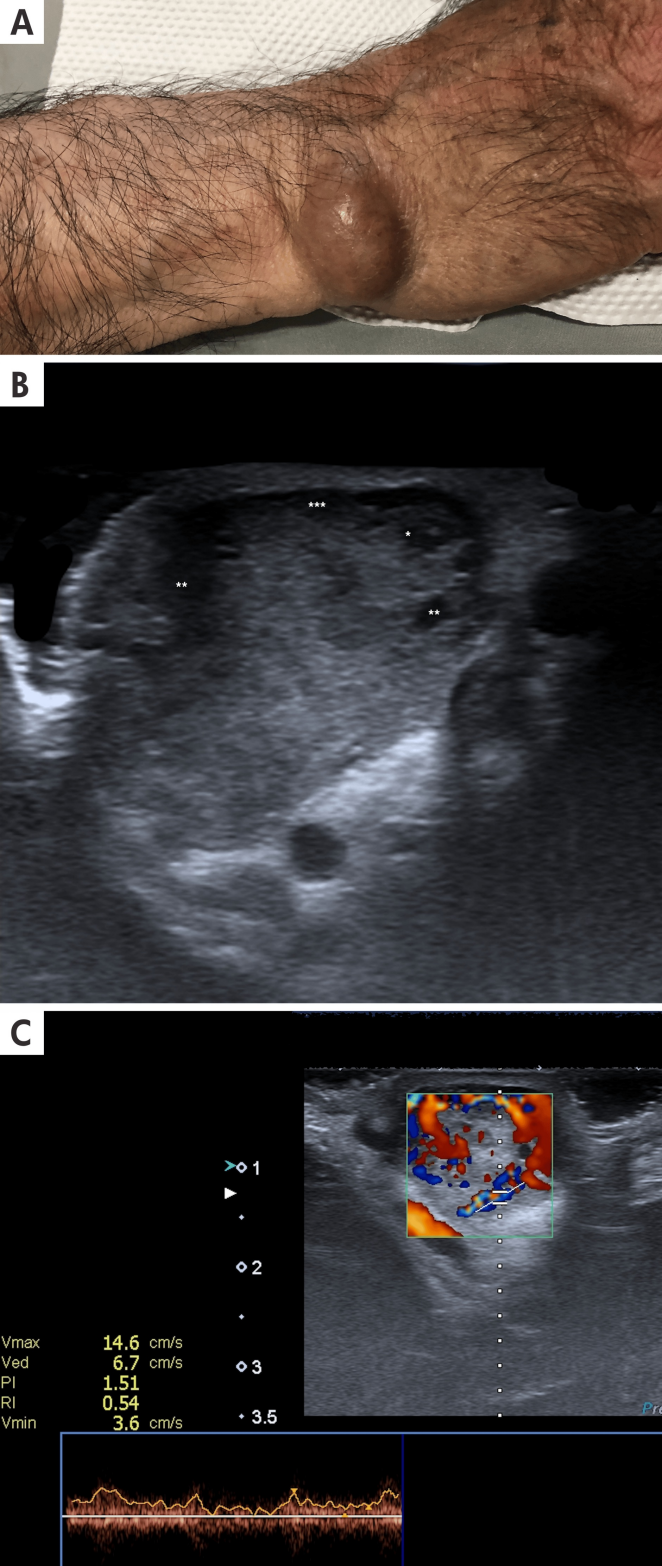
Dermatological ultrasound and clinical imaging of extradigital glomus tumor A: A 23-year-old male patient with a nodular, erythematous-violet, smooth, firm, oval, well-defined, well-demarcated, mobile tumor lesion, approximately 2 cm x 3 cm in size, located in the right metacarpal region. B: The longitudinal ultrasound view and grayscale ultrasound of the wrist region show an exophytic hypoechoic mass with well-defined borders; asterisks (*) show small hypoechoic serpentine capillaries inside the lesion. C: The spectral curve confirms the arterial origin of the vessel within the glomus tumor.
